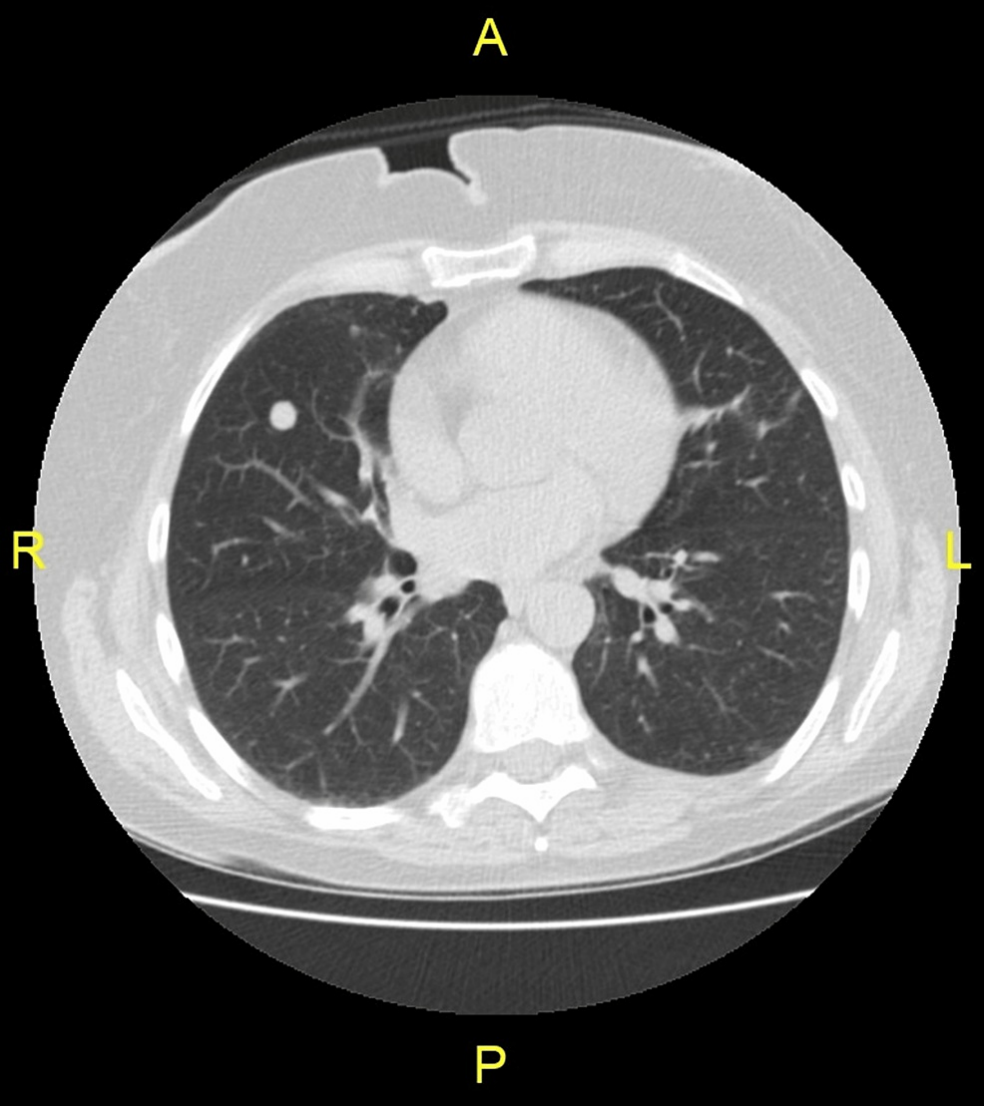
Chest CT findings of a 56-year-old female patient: axial non-contrast CT section through lung base 1 cm diameter right middle lobe nodule
